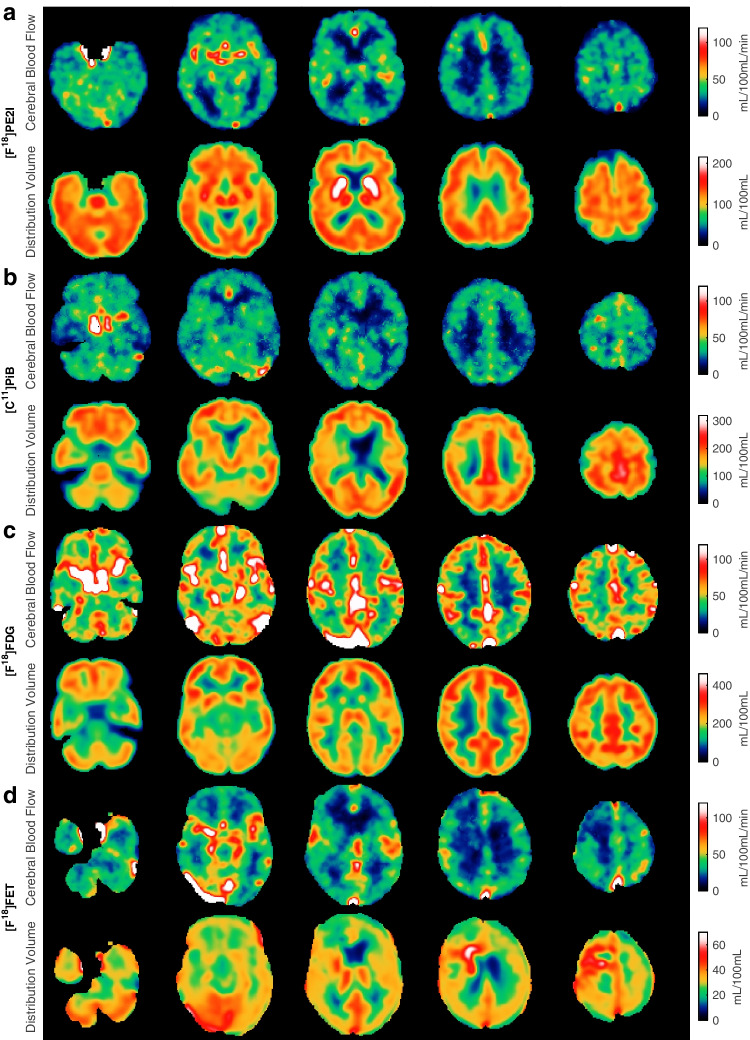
Each row shows the perfusion maps and volume of distribution maps for a [^11^C]PIB, b [^18^F]FE-PE2I, c 2-[^18^F]FDG and d [^18^F]FET for four different patients. Patient (a) had Alzheimer’s disease and pronounced beta-amyloid accumulation, and the CBF maps show typical parieto-temporal perfusion reduction (left-sided). Patient (b) was eventually diagnosed with major depression, and the CBF and volume of distribution maps were normal. Patient (c) had lung cancer with metastasis, but PET/CT of the brain did not disclose CNS involvement. Patient (d) had previously undergone surgery for brain cancer (glioblastoma), and the CBF maps show CBF reduction/no perfusion corresponding to the resection cavity, and the volume of distribution map shows abnormal frontal subcortical FET uptake, suggesting tumour recurrence. All images are shown in native orientation to avoid interpolation artefacts
